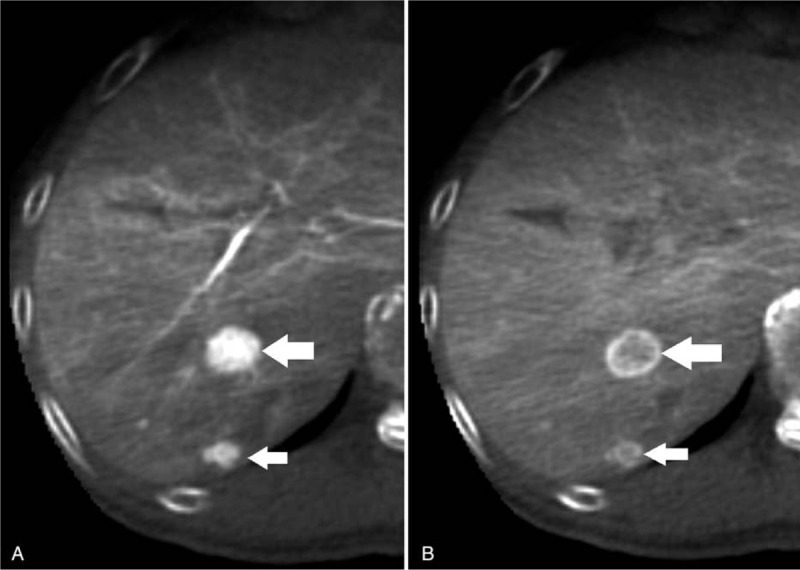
An 81-year -old man with hypervascular HCCs showing the typical CBCTHA finding. The first phase CBCTHA (A) demonstrated 2 hypervascular lesions with diameters of 1.6 cm and 0.8 cm (white arrow) in the subcapsular segment 8. The second phase CBCTHA (B) demonstrated washout of contrast material and rim enhancement, so called “corona enhancement” (white arrow). All readers rated them as HCCs with a high confidence level in both the first phase alone CBCTHA and the dual-phase CBCTHA.
